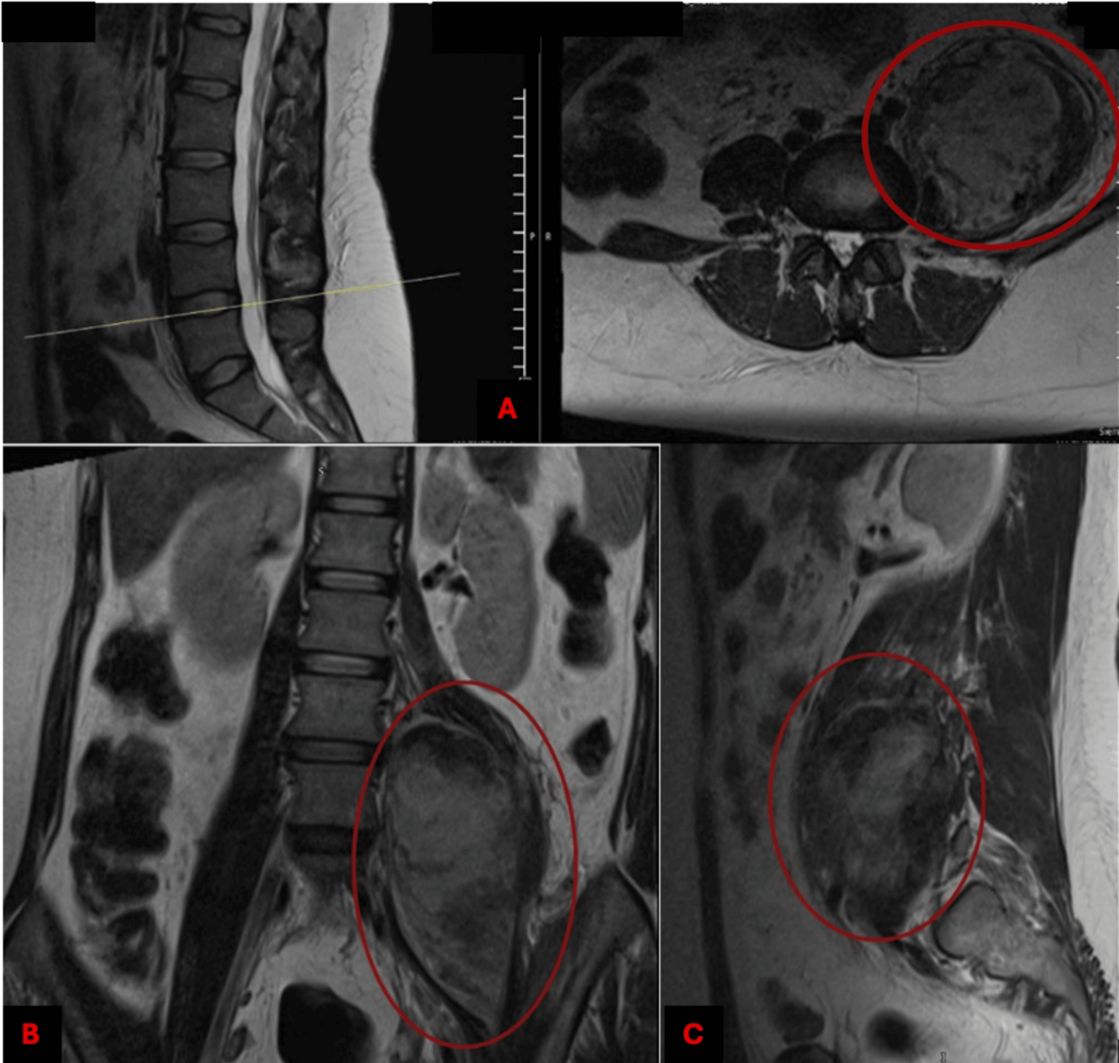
Hematoma in the iliopsoas visualized via magnetic resonance imaging (MRI) after drainage at the one-month follow up. Contrast MRI in a T2 sequence showing a decrease in the initial volume to 352 cc. (A) Cross-section; (B) Coronal section; (C) Sagittal section. Cc: cubic centimeters
